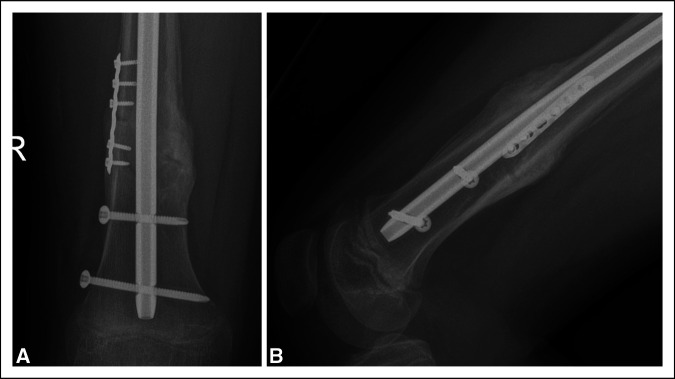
Robust bridging fracture callus seen in AP (A) and lateral (B) radiographs of the distal femur, suggestive of secondary healing of the fracture.
